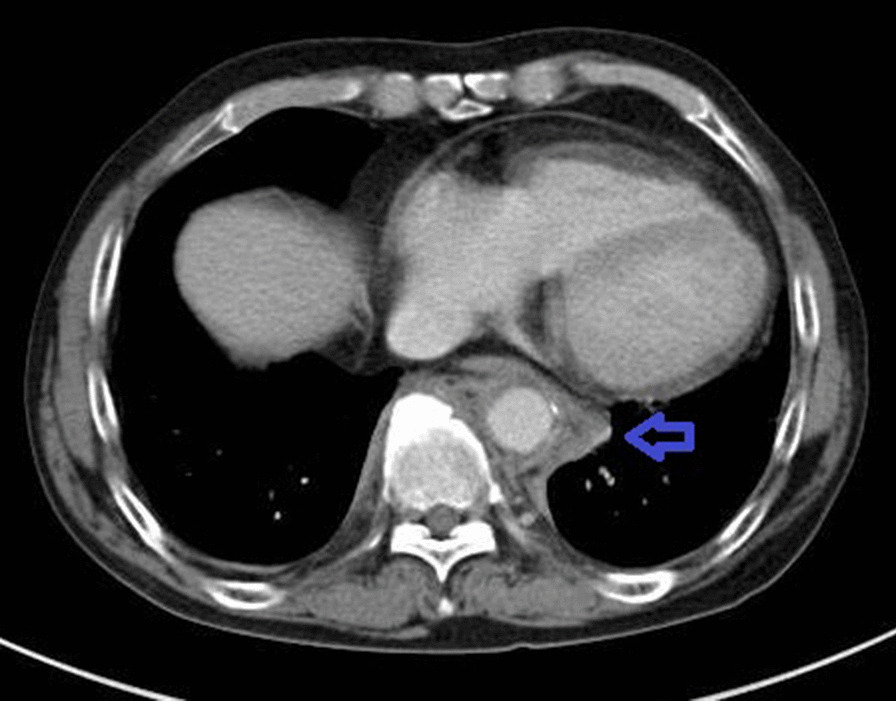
Computed tomography examination of the abdomen showing thickened and edematous areas surrounding the aorta (blue arrow)
